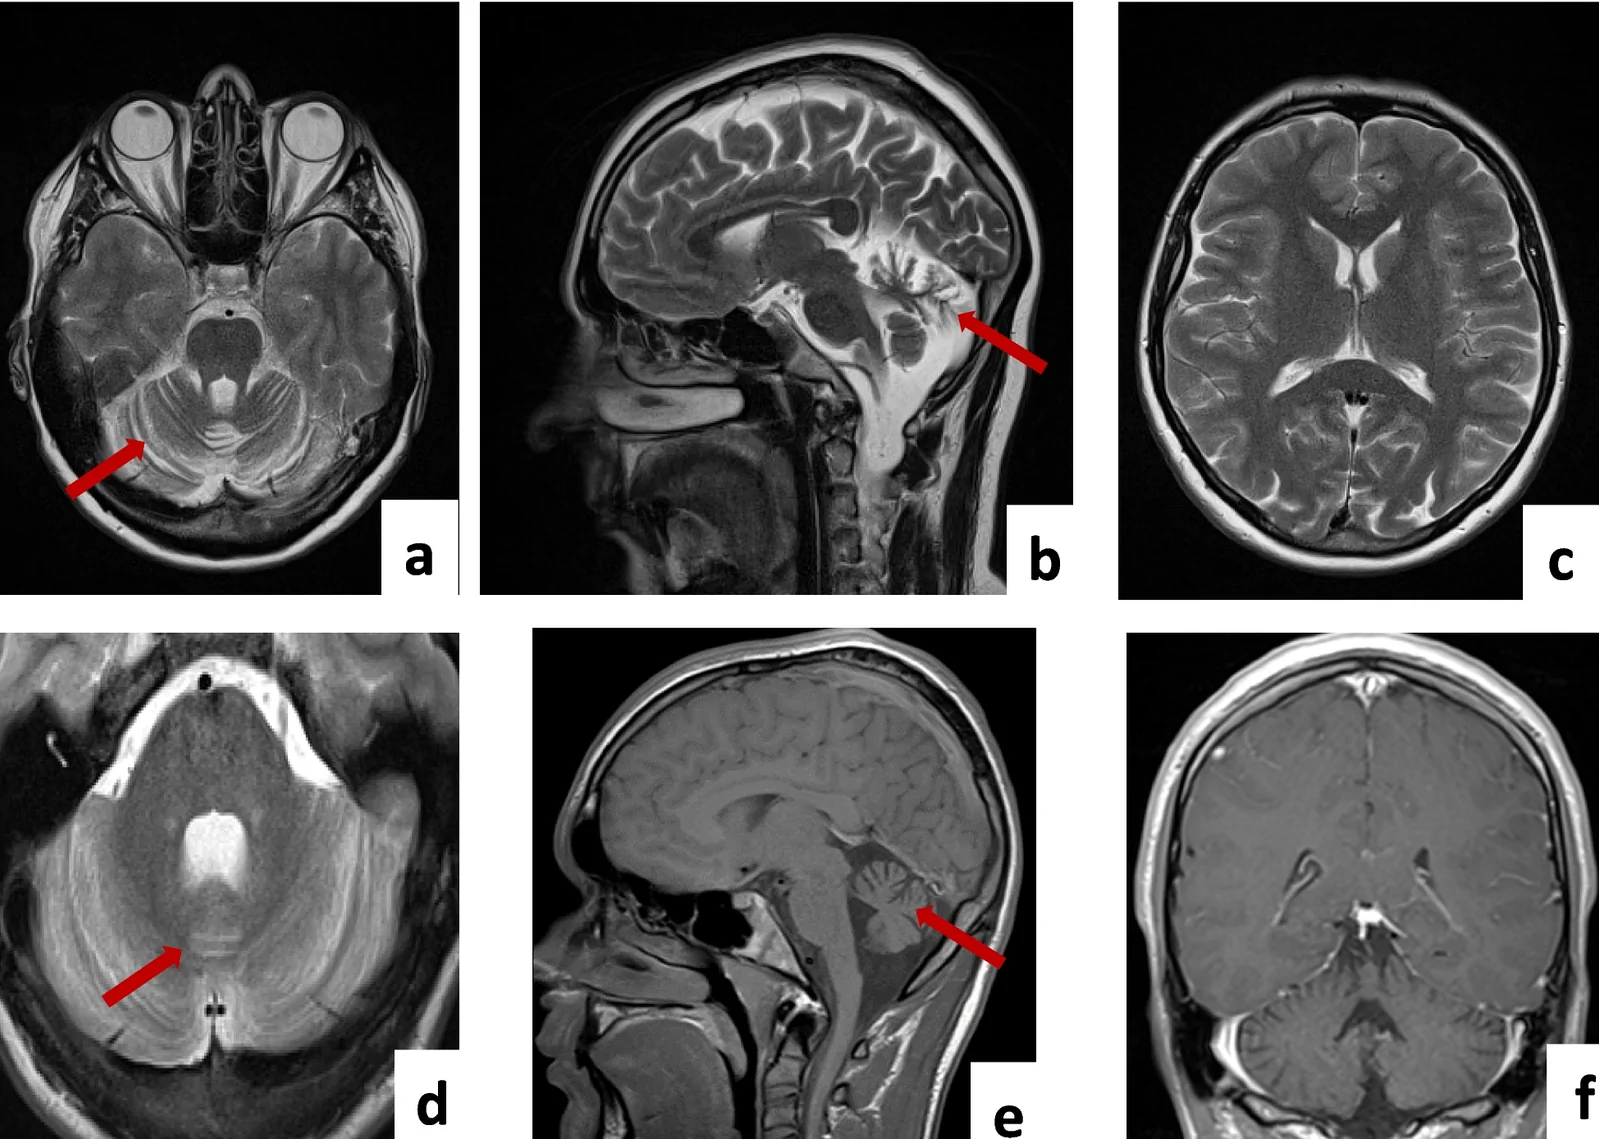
Brain MRI. T2-weighted images, cerebellum atrophy on transverse a and sagittal b images, and normal supratentorial brain structures c of Patient 1. Transverse T2-weighted image d, mid-sagittal T1-weighted image shows moderate atrophy of the vermis e, coronal T1-weighted image confirms atrophy of the vermis and shows atrophy of the cerebellar hemispheres f of Patient 2
